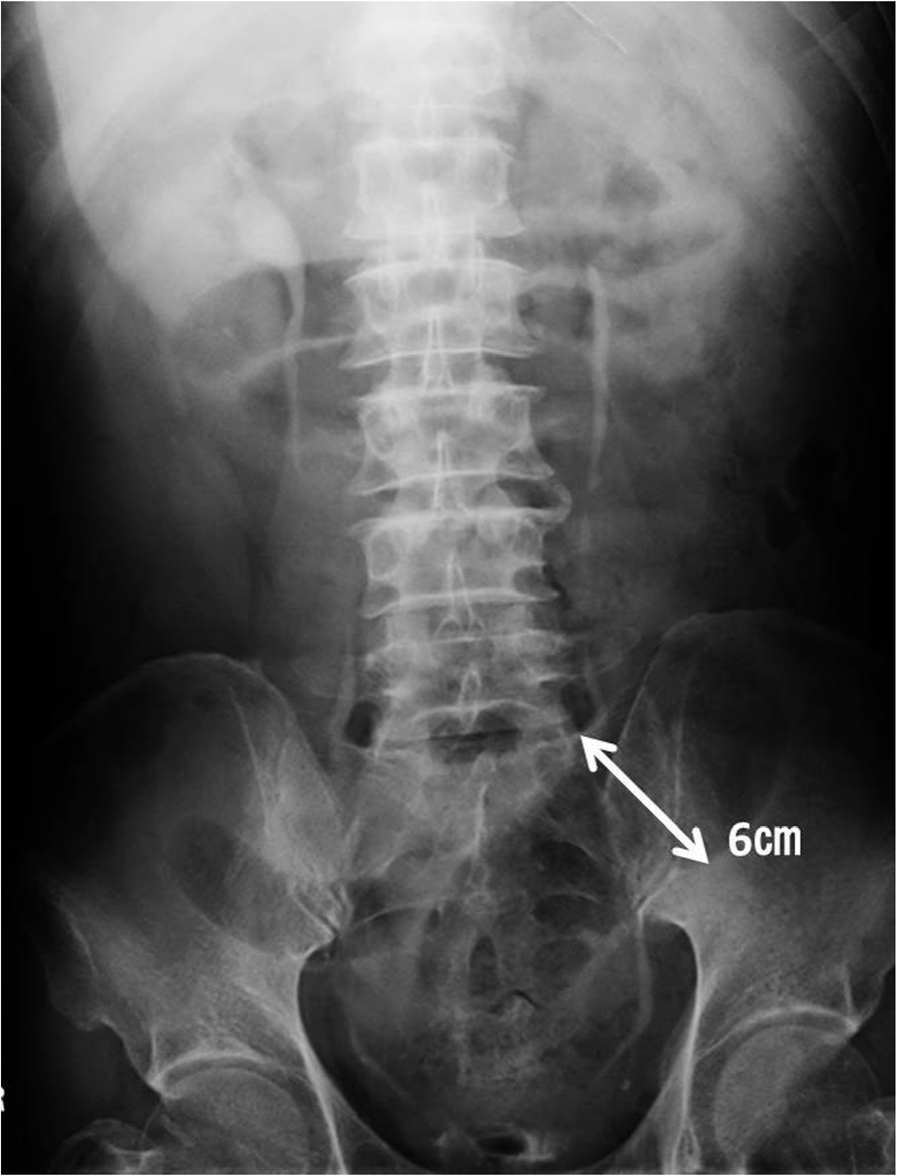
Abdominal X-ray examination revealed 6-cm dilatation of the sigmoid colon with fecal impaction
